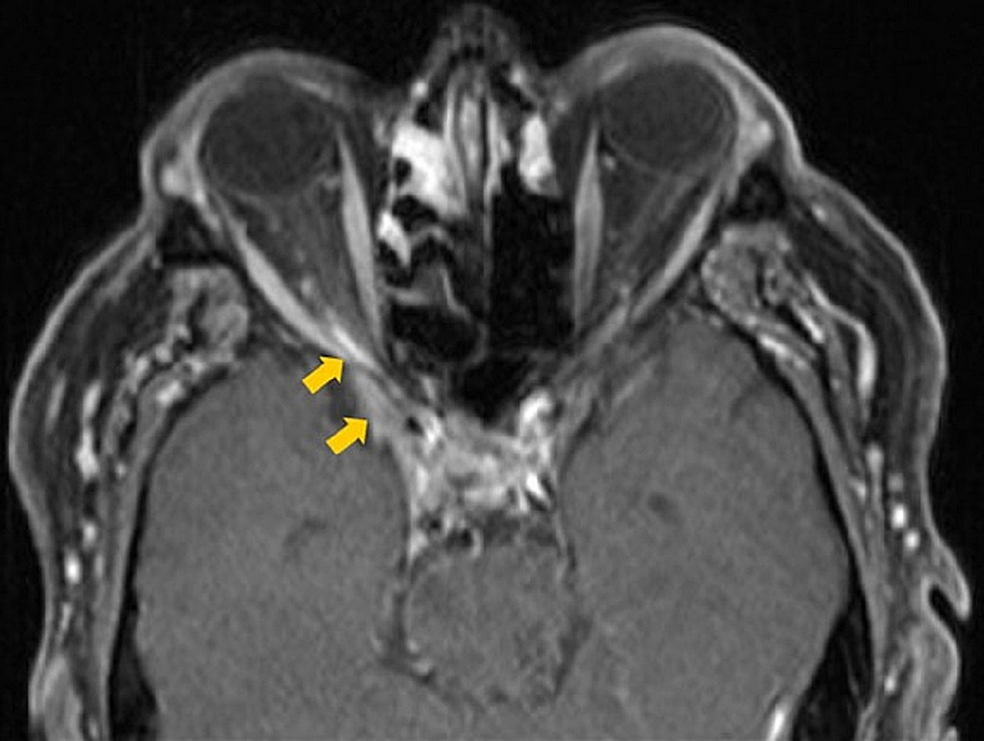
Initial axial contrast-enhanced T1-weighted MRI The image is demonstrating enhancement of the right posterior orbital apex (yellow arrow).
